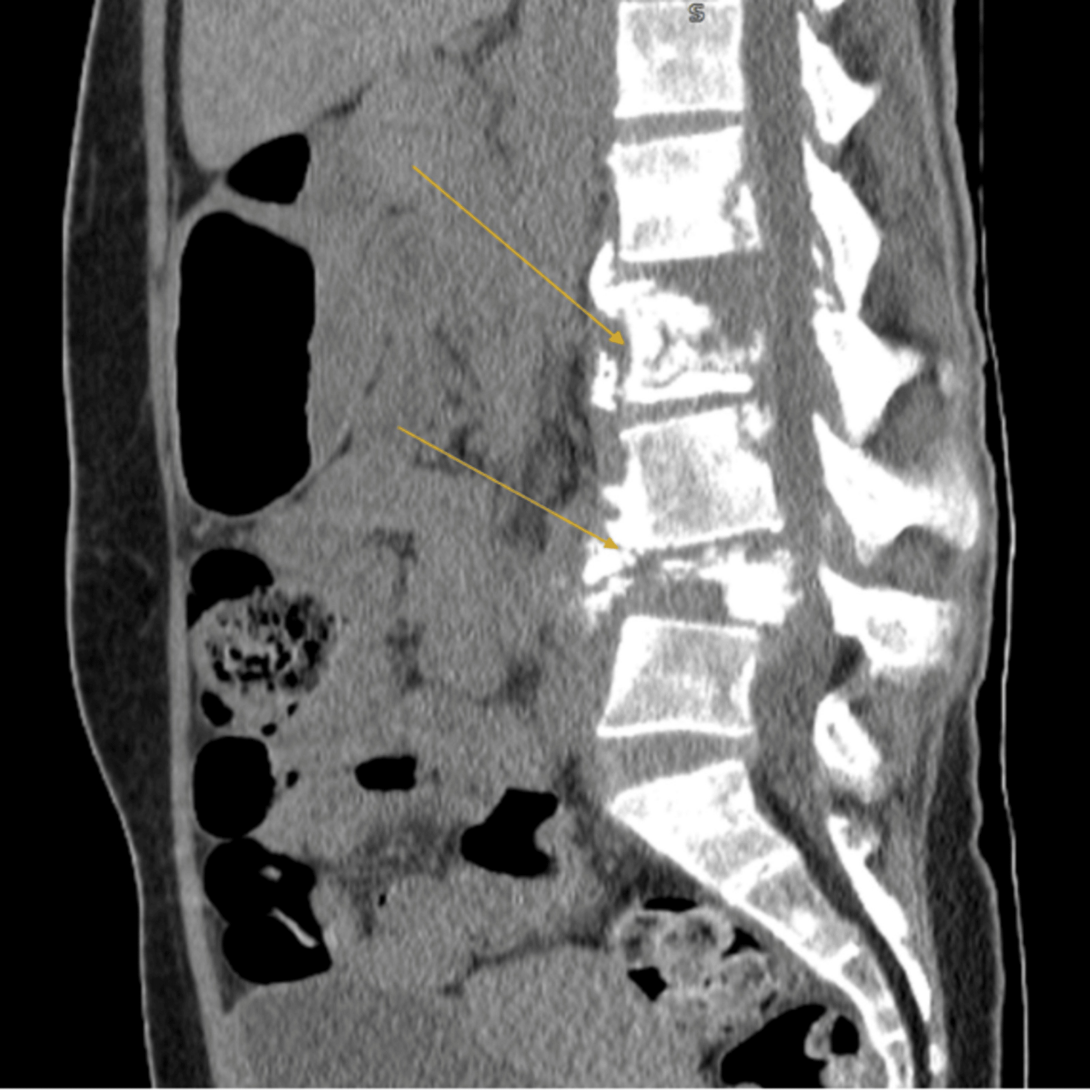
MRI scans of the cervical, thoracic, lumbar, and sacral spine highly suggestive of tuberculous spondylitis, with multilevel spinal canal compromise from T11 to L5
